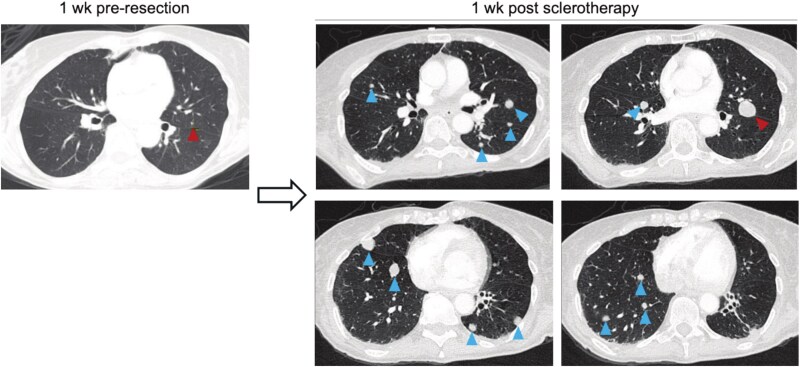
Development of metastatic disease throughout patient’s treatment course. CT chest with contrast is depicted after Moh’s and prior to radical left neck resection (left) and follow-up imaging 1 week after sclerotherapy. Red arrow marks the initial metastatic lesion. Blue arrows indicate subsequent metastases that developed following surgical/procedural interventions.
